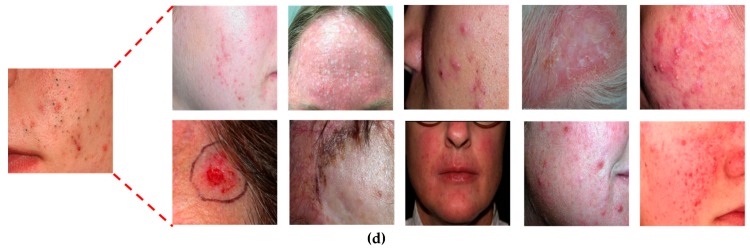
Examples of good retrieval performance of our proposed system for input query image. (a) colonography CT; (b) liver CT; (c) upper endoscopy; (d) facial acne.
